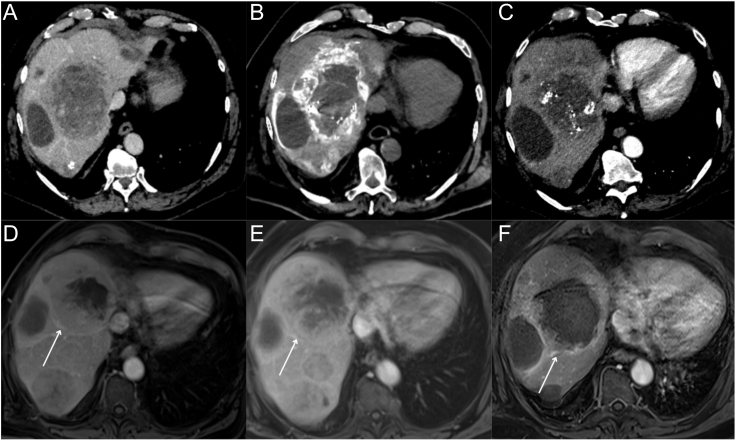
Pre- and post-operative imaging of a c-TACE procedure in a 70-year-old patient with pulmonary NET. (A) The CT scan of the liver in axial plane at portal phase 6 weeks before c-TACE procedure shows a voluminous heterogeneous metastasis of the hepatic dome and a scar of a lesion previously treated in segment VIII. (B) Preoperative cone-beam CT reveals peripheral arterial enhancement of the targeted metastasis with a devascularized center. (C) The follow-up CT scan of the liver in axial plane at arterial phase at 5 months shows a complete peripheral devascularization of the targeted metastasis, with the presence of intra-tumoral Lipiodol. (D) MRI in axial plane, T1-weighted image at arterial phase after gadolinium injection 4 weeks before c-TACE, showing the fleshy portion of the metastasis. (E) The follow-up MRI in axial plane, T1-weighted image at arterial phase at one month reveals a partial devascularization. (F) The follow-up MRI in axial plane, T1-weighted image at arterial phase at 6 months shows a complete devascularization.
